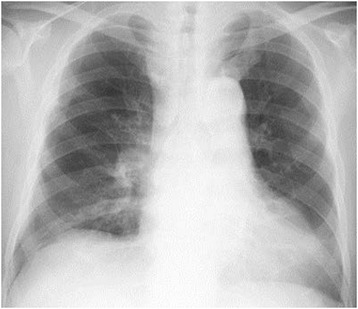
Chest X-ray showing mild cardiomegaly without pulmonary congestion
